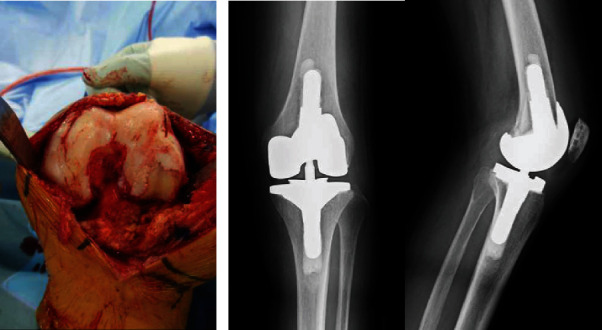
TKA. (a) Intraoperative photograph showing that the cartilage of the lateral joint surface of her left knee was scooped out and eburnated partially. (b) Postoperative radiographs of left TKA using a semiconstrained prosthesis.
